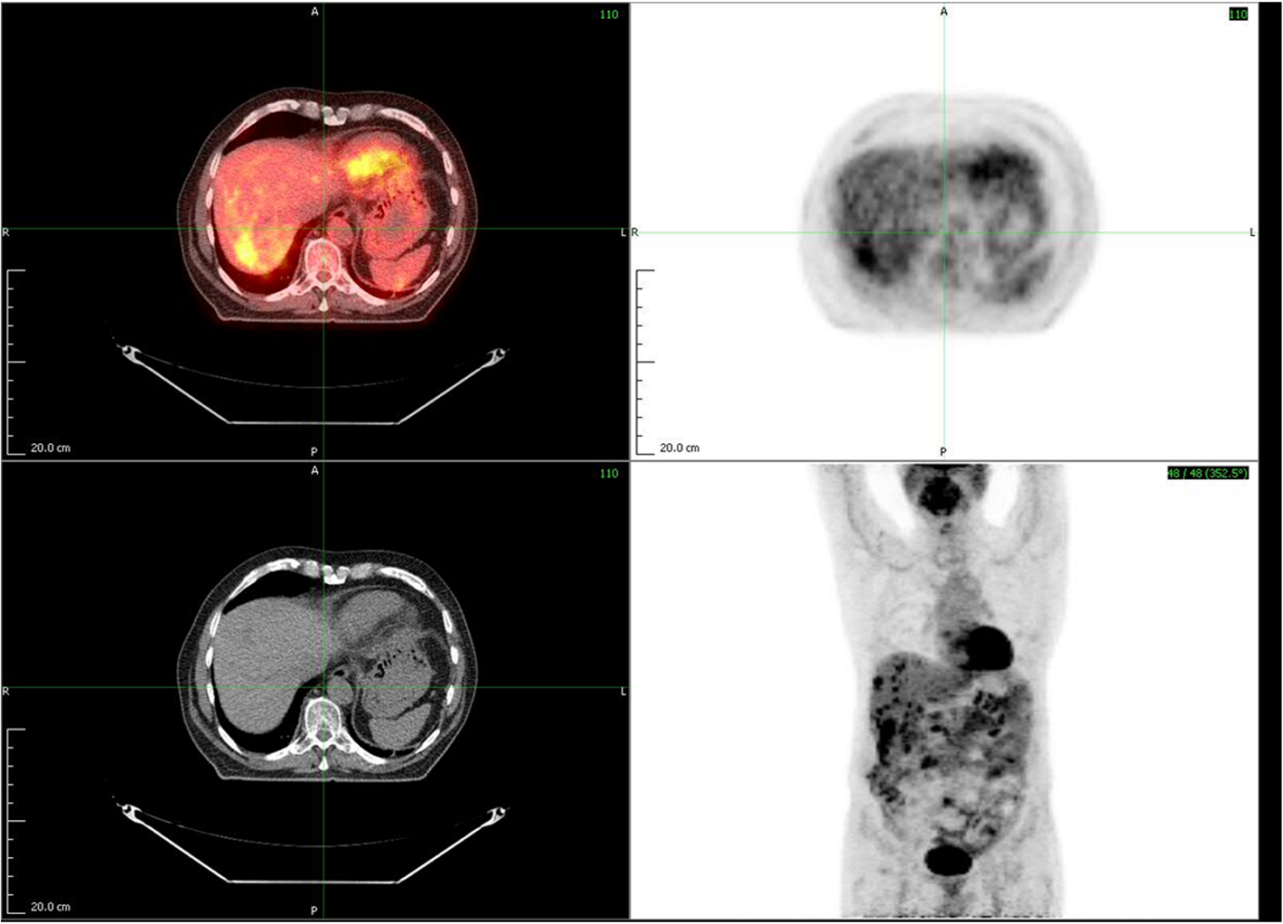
Positron emission tomography-computed tomography scan showing multiple liver metastasis immediately post-pancreaticoduodenectomy and prior to first-line palliative chemotherapy.
